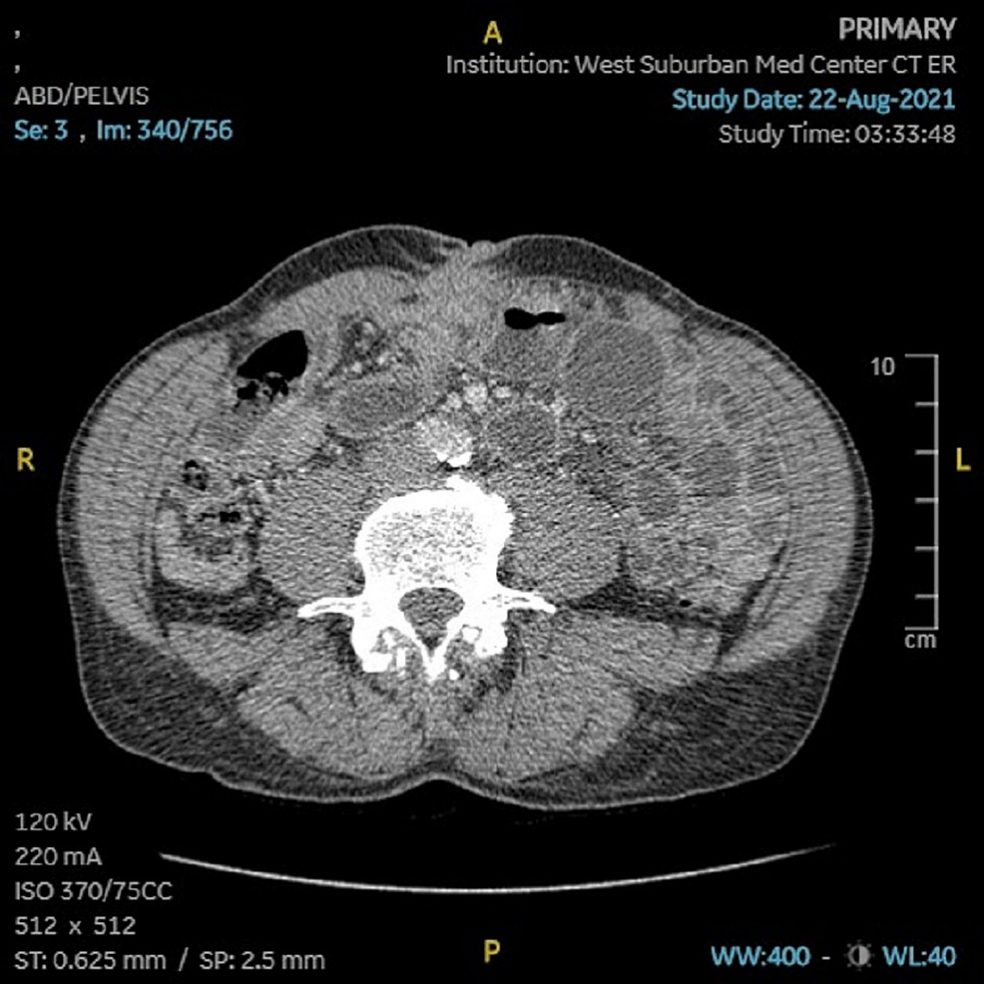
Abdominal CT showing soft tissue thickening deep to the umbilicus.
